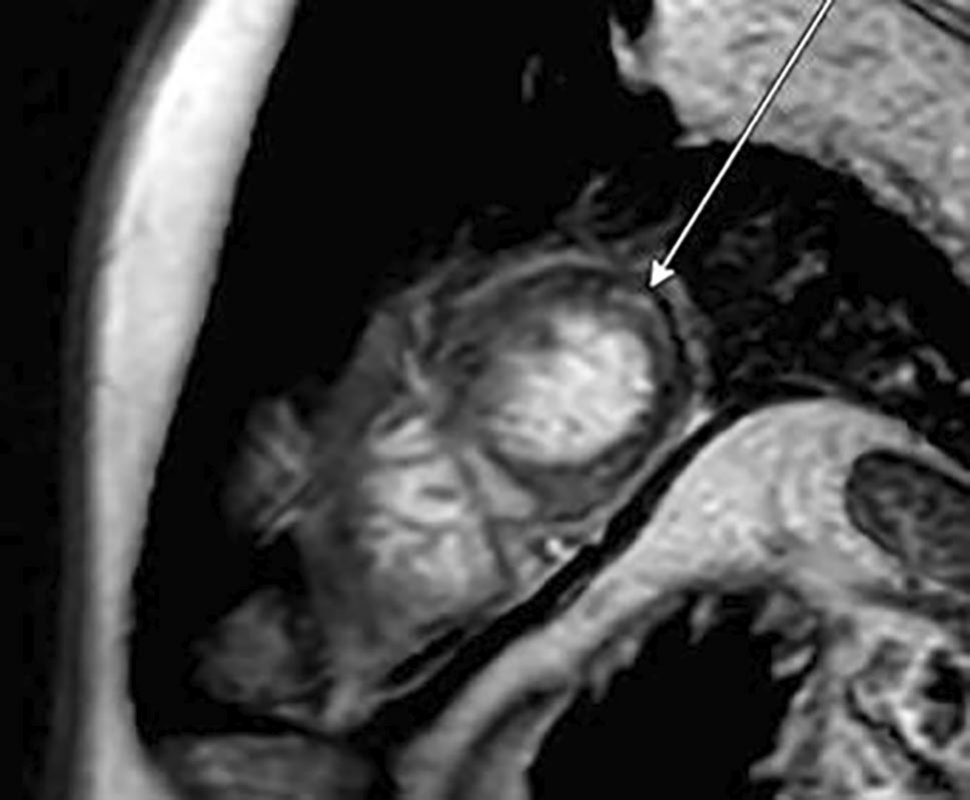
Cardiac magnetic resonance imaging (MRI). Phase-Sensitive Inversion Recovery Turbo Field Echo (PSIR TFE) sequences in short axis view show midmyocardial late gadolinium enhancement in the apical lateral wall of the left ventricle (arrow)
